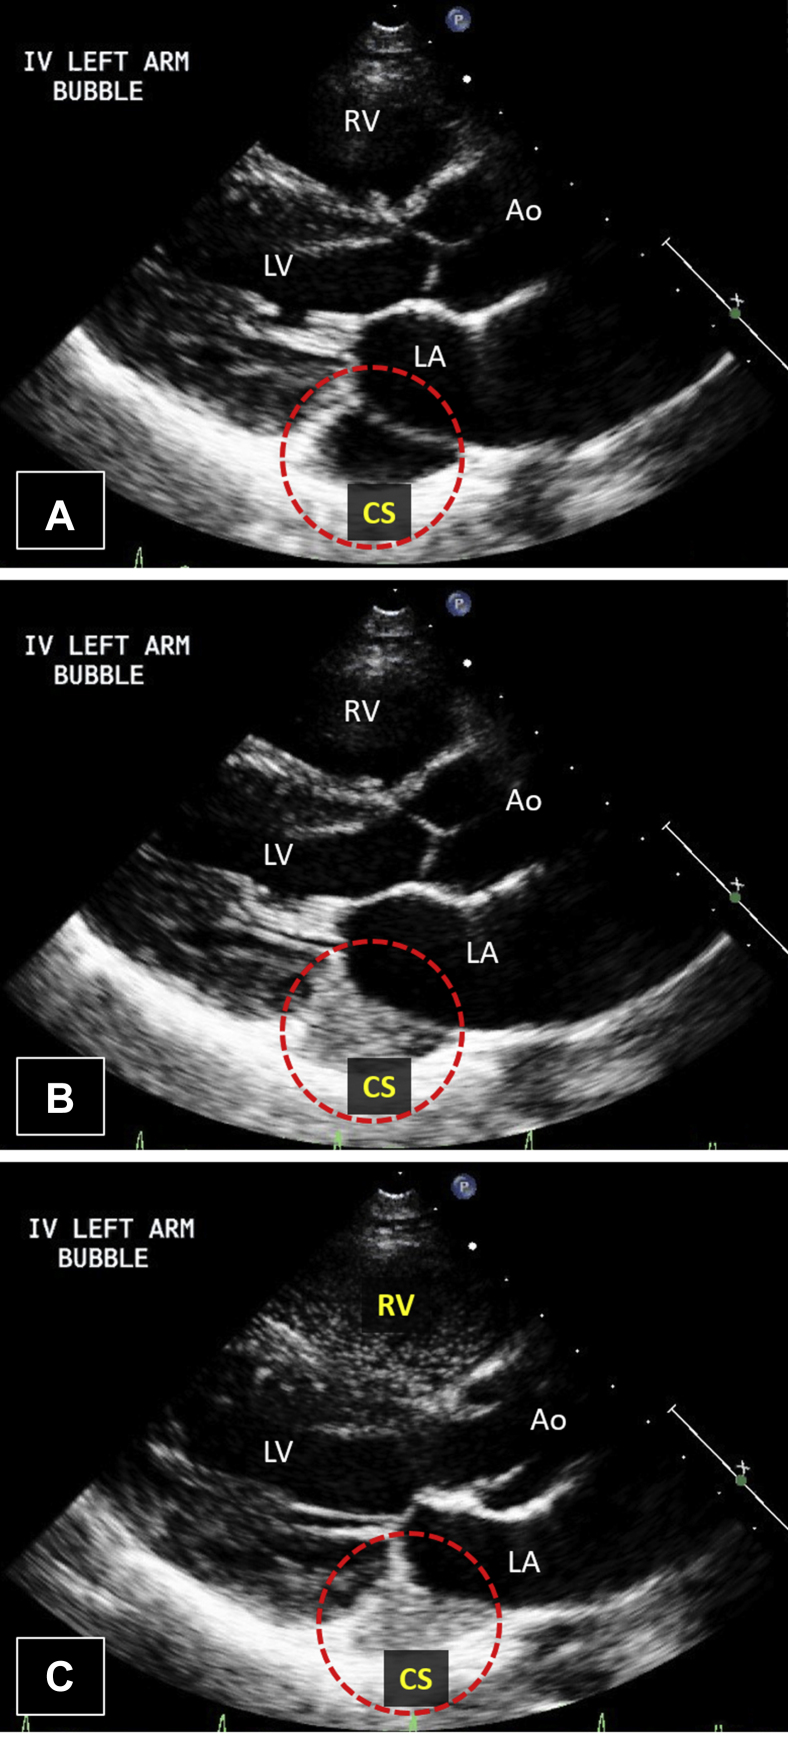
Case 1: persistent L-SVC. Transthoracic echocardiogram in parasternal long-axis view with intravenous agitated saline injection in left arm. The presence of a dilated CS on TTE (A) suggests a venous anomaly. After injection of agitated saline into the patient's left arm, the dilated CS opacifies first (B), followed by the right heart (C). This opacification pattern is consistent with the diagnosis of a persistent L-SVC. Video 1 corresponds to this figure. *Ao*, Aorta; *CS*, coronary sinus; *RV*, right ventricle.
